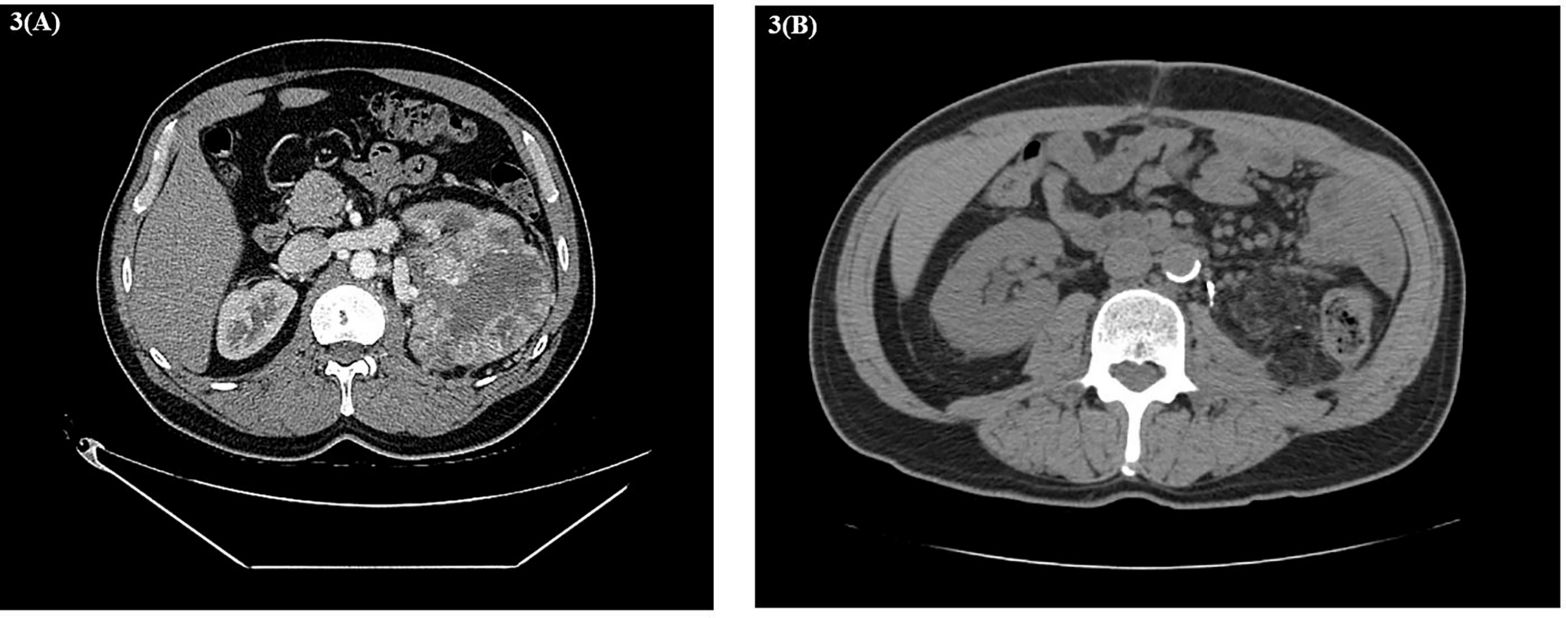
(A) Baseline CT abdomen and pelvis with left renal cell carcinoma. (B) CT abdomen 2 months after left radical nephrectomy.
